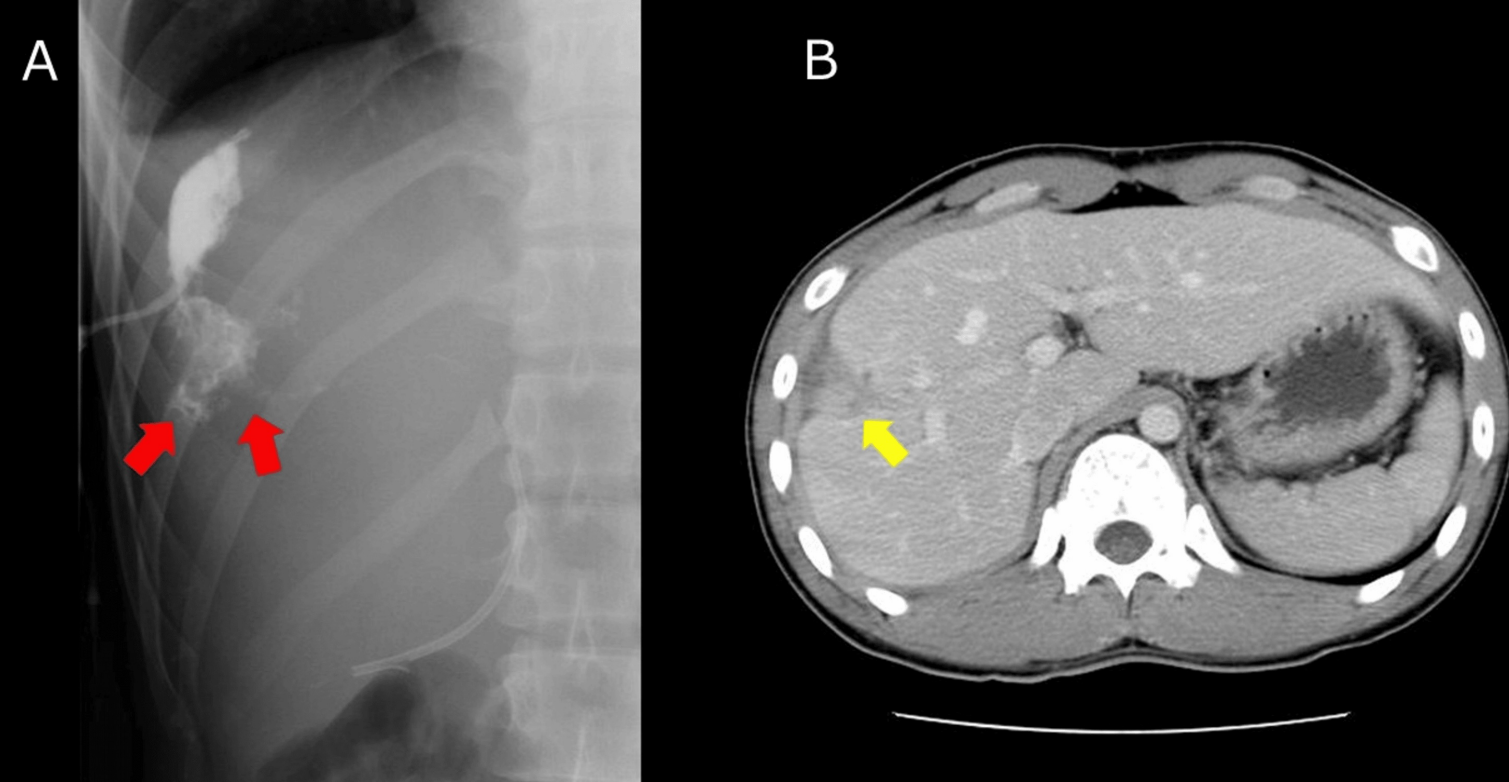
Leak closure and follow-up (A) Tubography via the percutaneous biloma drain on hospital day 40 shows focal pooling of contrast within the fistulous tract only (red arrows) without opacification of the intrahepatic bile ducts, indicating no residual communication. (B) Follow-up contrast-enhanced CT demonstrates complete resolution of the biloma; the site adjacent to the prior laceration is indicated (yellow arrow).
